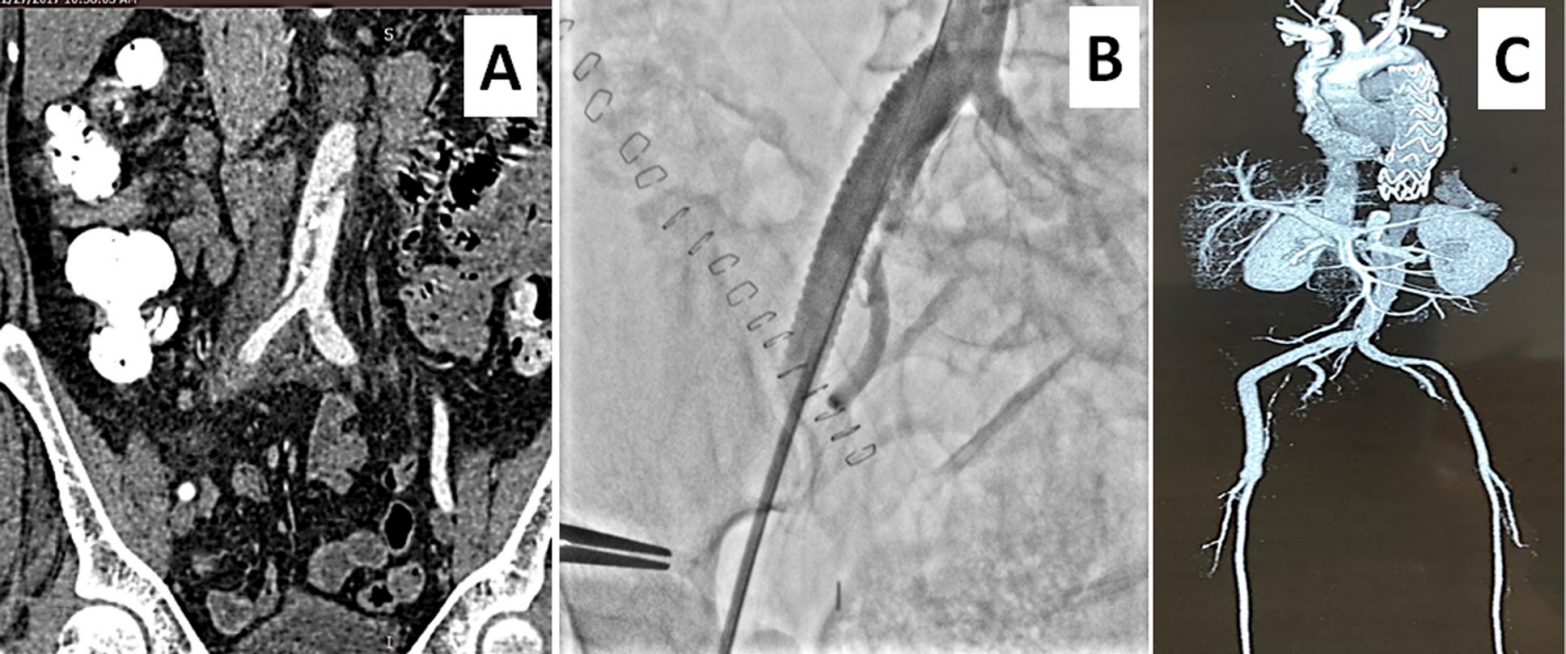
Coronal reconstructed CT image (A) of case 8 showed type-B aortic dissection extending up to left CIA and diseased right CIA. A contrast angiogram showed anastomosed conduit at the level of aortic bifurcation (B). A follow-up, 3-dimensional volume-rendered CT angiographic image showed patent aorto-right femoral bypass graft, diseased right CIA and patent descending thoracic aorta stent-graft (C).
